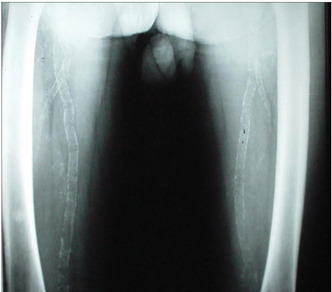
Femoral radiograph showing vascular calcifications of the femoral vessels.
